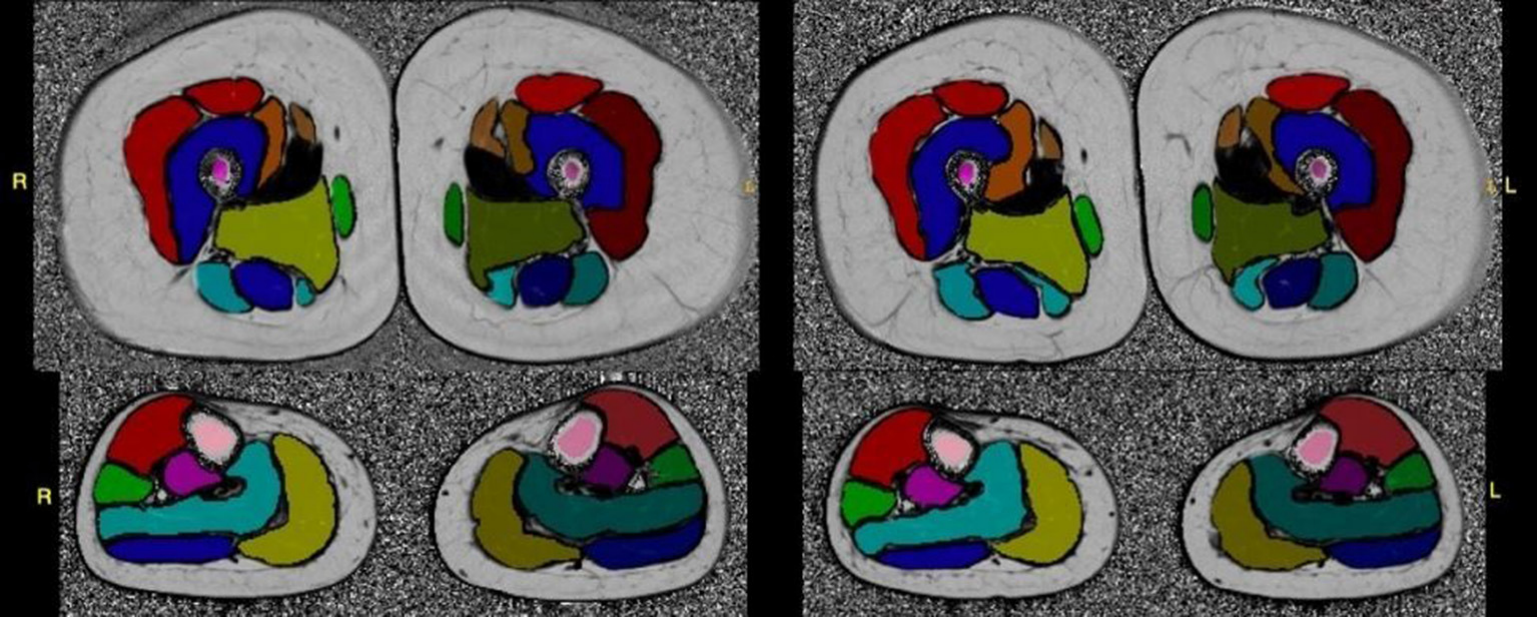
Test-retest fat-fraction map images from an example healthy volunteer for the calculation of reproducibility metrics. Images were acquired at two time points, 2 weeks apart. The fat fraction map was calculated from three-point Dixon acquisitions using the MRI study protocol, and shown with the placement of the whole muscle ROIs overlaid on the slice. All images were windowed to the same range for display (0–100%).
